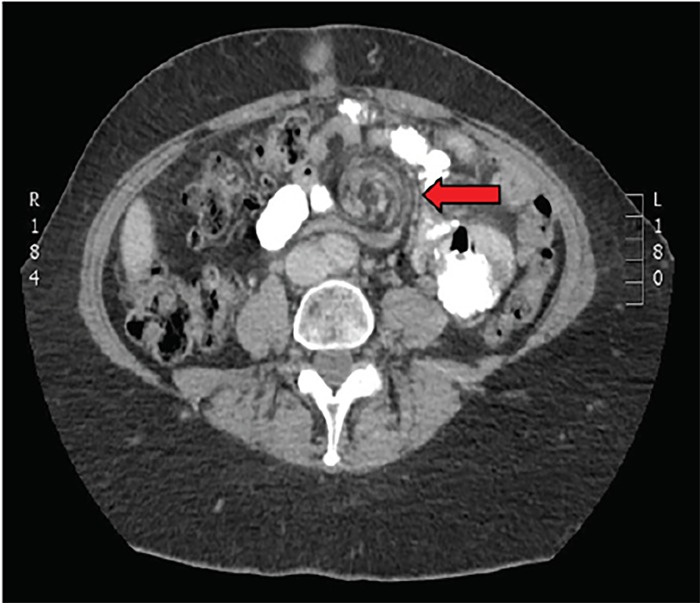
Axial computed tomography of the abdomen shows “swirl sign” indicative of internal hernia (red arrow).
